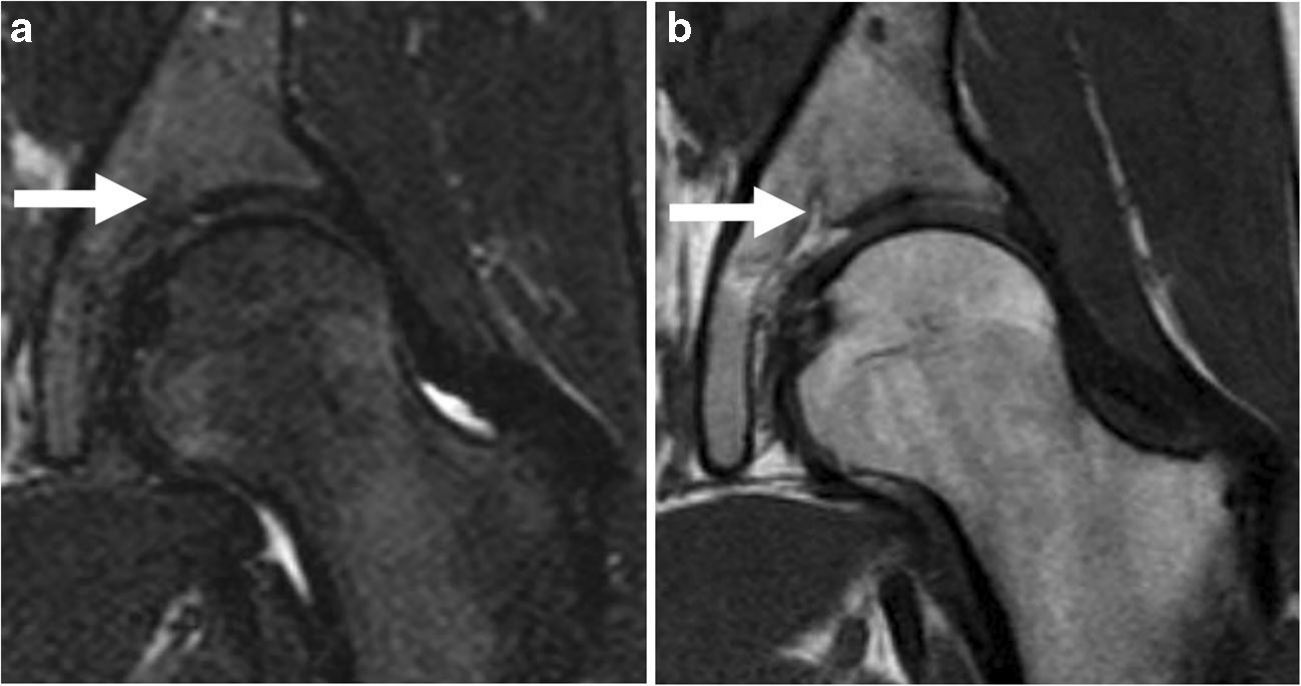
Seventeen-year-old female. Coronal short tau inversion recovery (a) and coronal T1-weighted (b) MR images show fat-like findings within the SARN (arrows) of the left hip, classified as a SARN type-2
